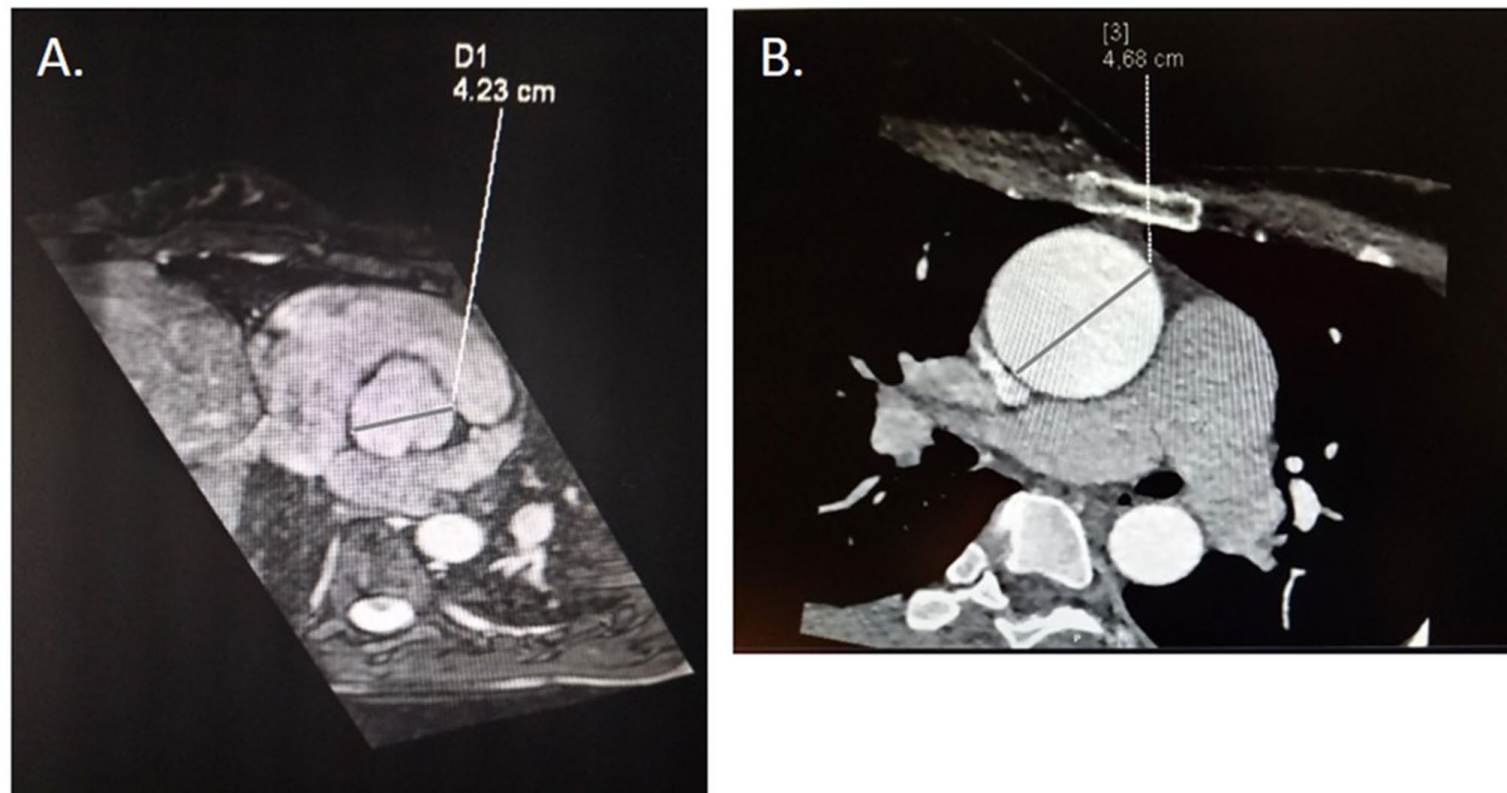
Ascending aortic aneurysms. (A): Magnetic resonance aortography of the first parturient. (B): Multislice computed tomography aortography of the second parturient
